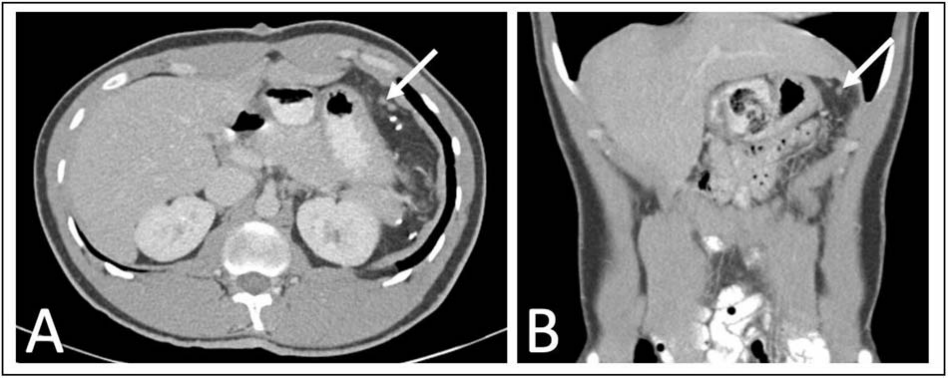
One year post operative abdominal CT scan (A) axial and (B) coronal abdominal CT images showing several sub-centimeter nodules in the post-splenectomy bed (arrows).
